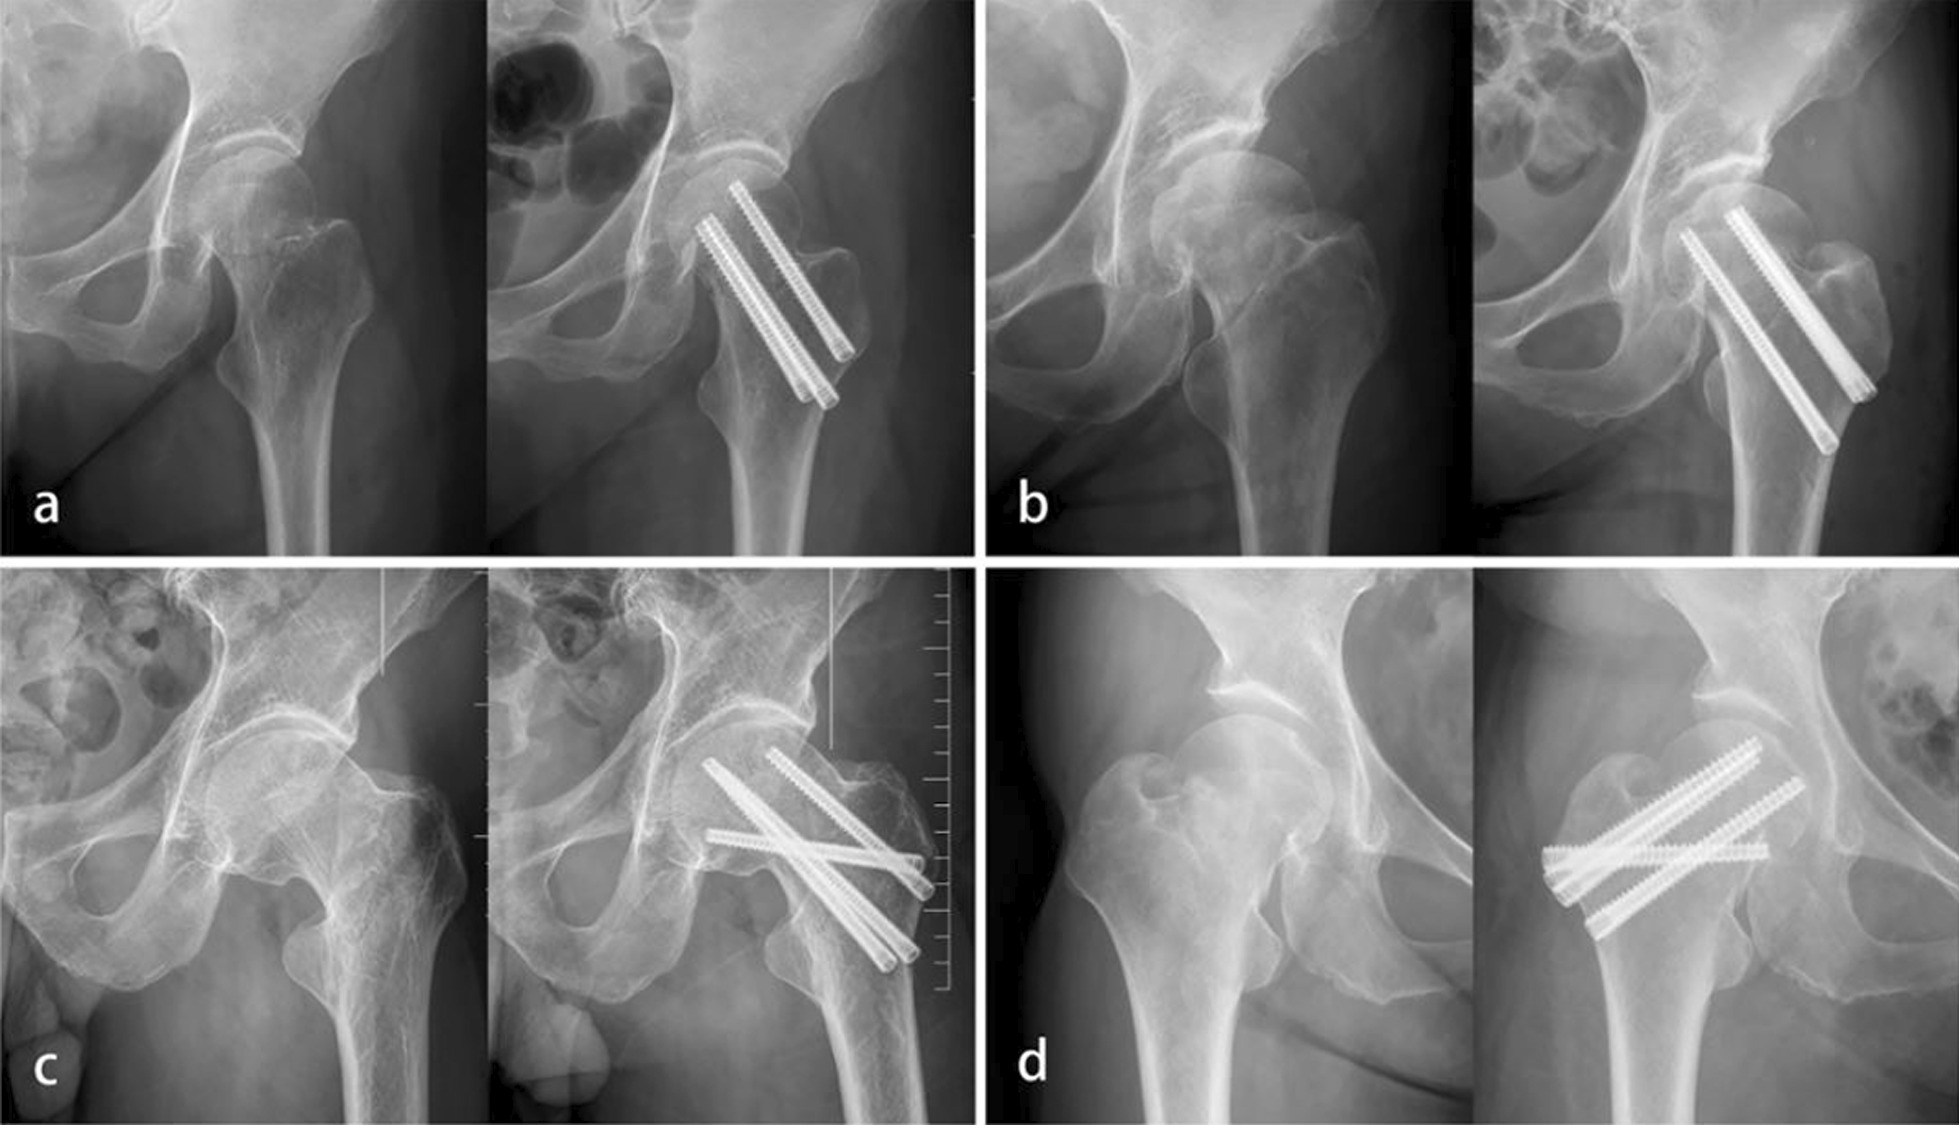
Preoperative and postoperative anterior posterior radiographs of femoral neck fracture treated with fully threaded cannulated compression screws. a Fixed by three screws in a triangle configuration. b Fixed by three screws in an inverted triangle configuration. c Fixed by four screws in a triangle configuration. d Fixed by four screws in an inverted triangle configuration
